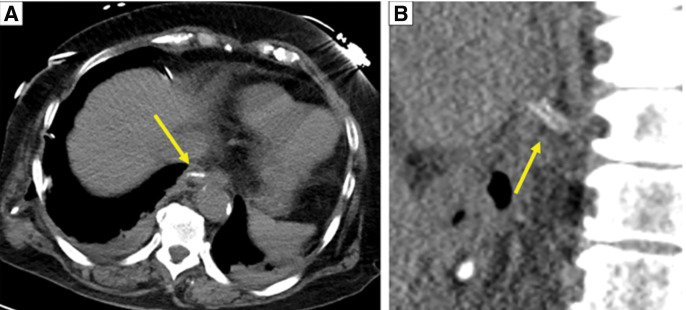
CT immediately after surgery. (A) Axial view and (B) sagittal view. A double-linear hyperabsorptive structure is observed near the esophagectomy site (yellow arrows).
